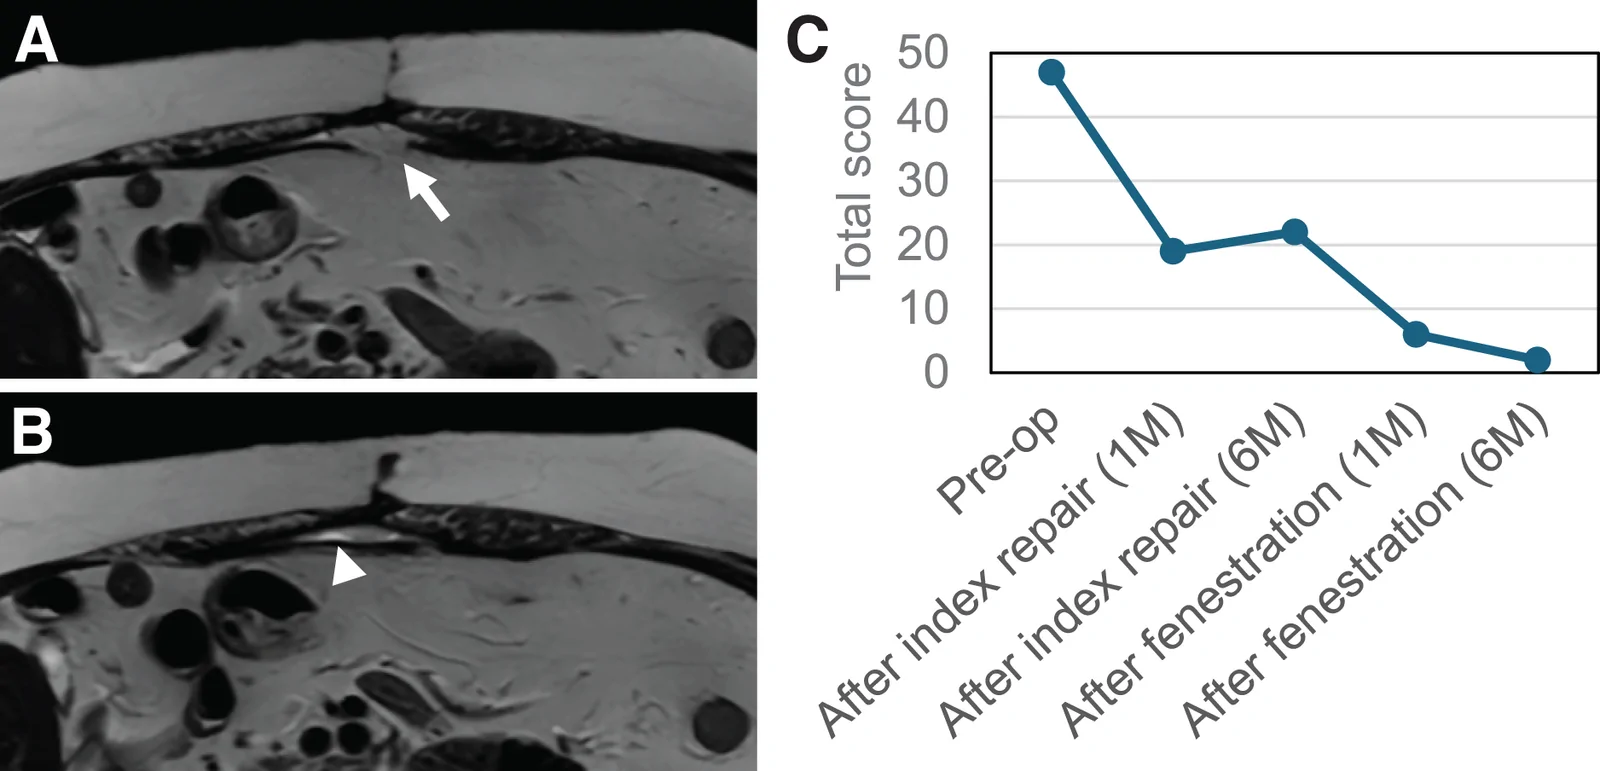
Postoperative MRI and QOL outcome after laparoscopic fenestration. (A) Postoperative axial T2-weighted MRI demonstrating omental protrusion through the mesh fenestration site (arrow) without bowel incarceration. (B) A small residual T2-high fluid space (arrowhead) adjacent to the omental interposition with substantial overall shrinkage of the chronic seroma cavity compared with the preoperative findings. (C) Transition of the total European Hernia Society QOL score demonstrating transient deterioration after the index repair and marked improvement after laparoscopic fenestration.
